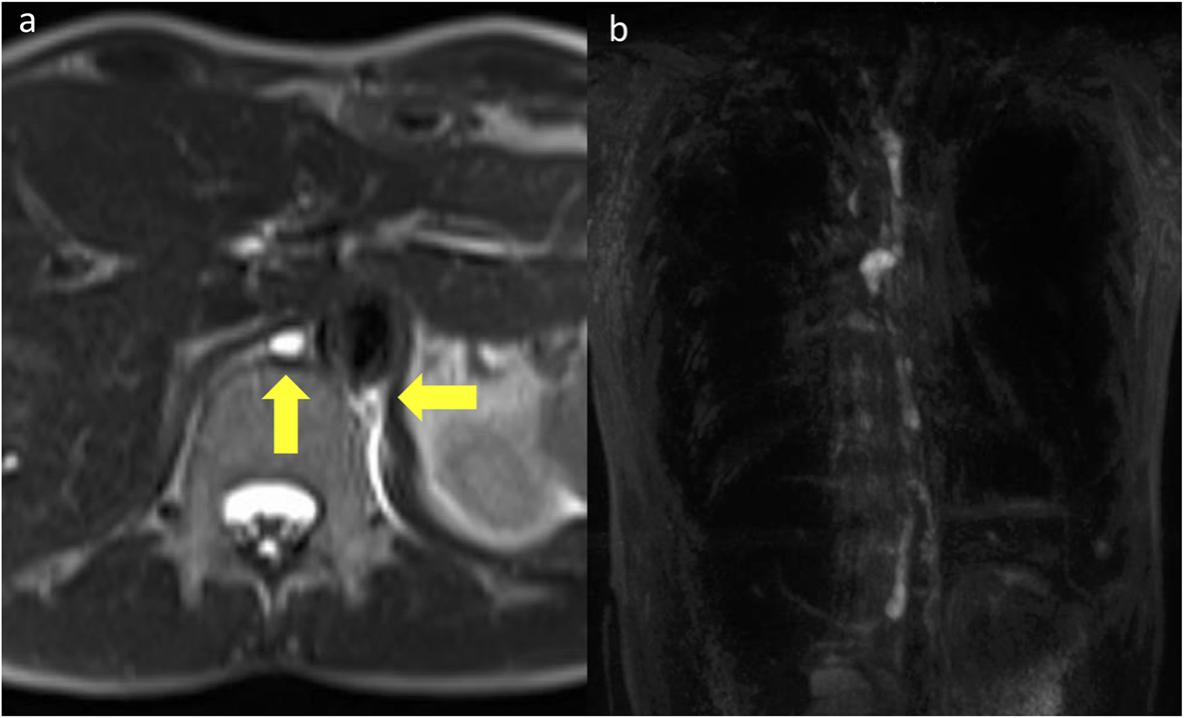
a MRI 10 days after admission revealed two thoracic ducts running through the thoracic cavity. b Coronal imaging of MRI
